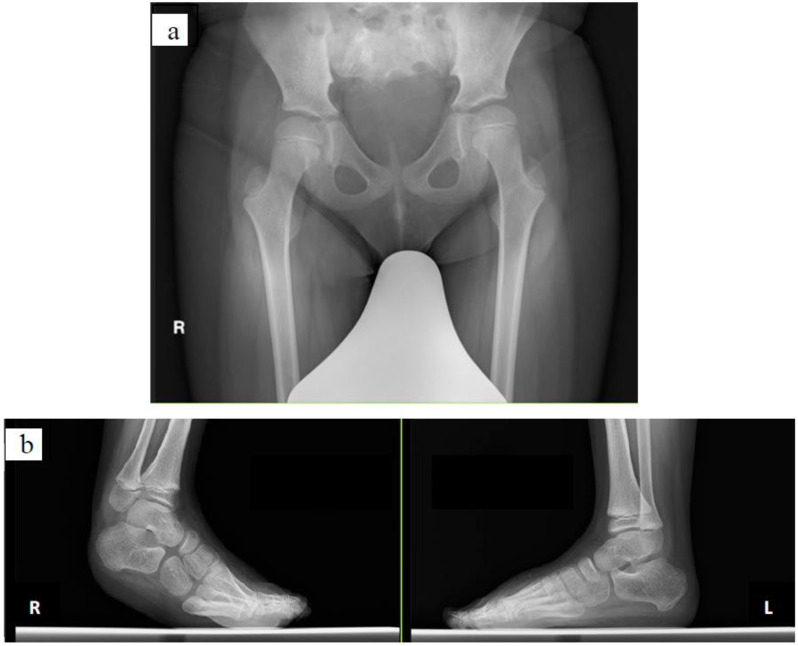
Radiographs of case 1: (a) pelvic radiograph and (b) radiograph of the feet.
